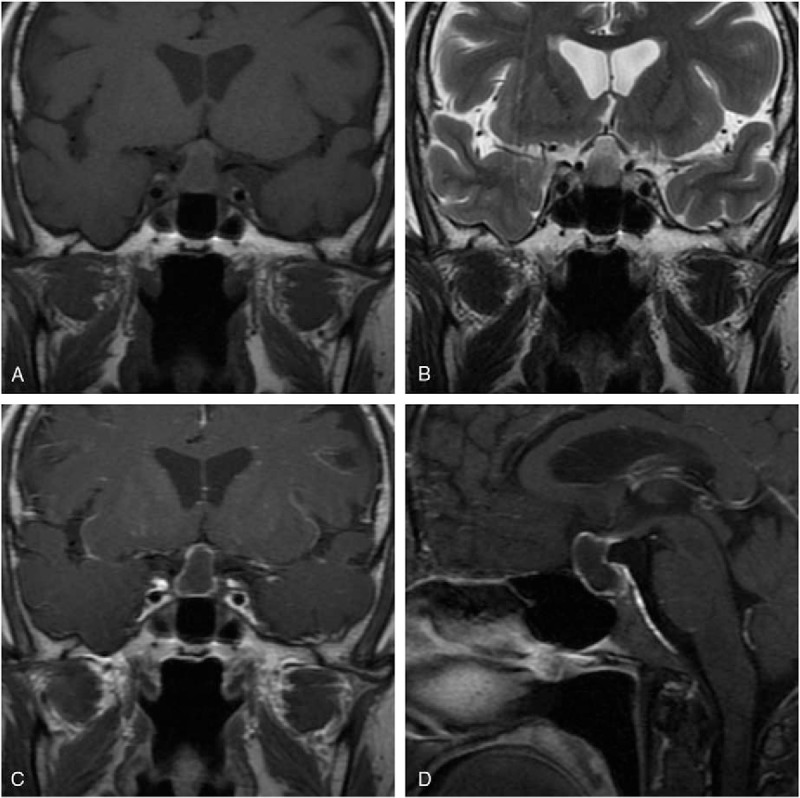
Preoperative MRI of showed the sellar and suprasellar entity. (A) Coronal T1WI; (B) coronal T2WI; (C) coronal-enhanced T1WI; (D) sagittal-enhanced T1WI. MRI = magnetic resonance imaging, T1WI = T1-weighted image, T2WI = T2-weighted image.
